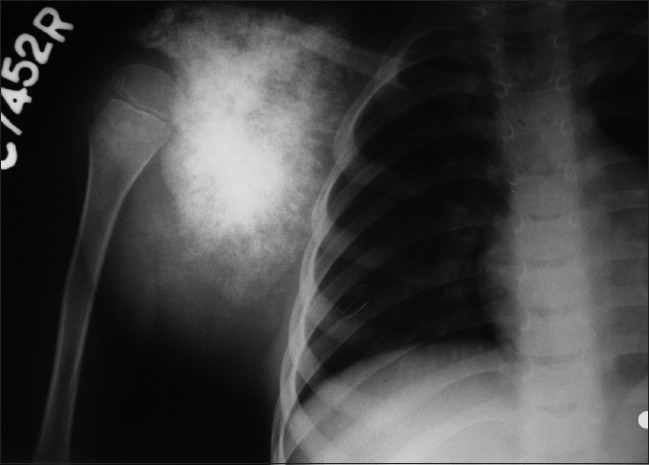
Pre-treatment radiograph of right shoulder showing a sclerotic lesion of scapula with loss of definition of margins of scapula and soft tissue mass
